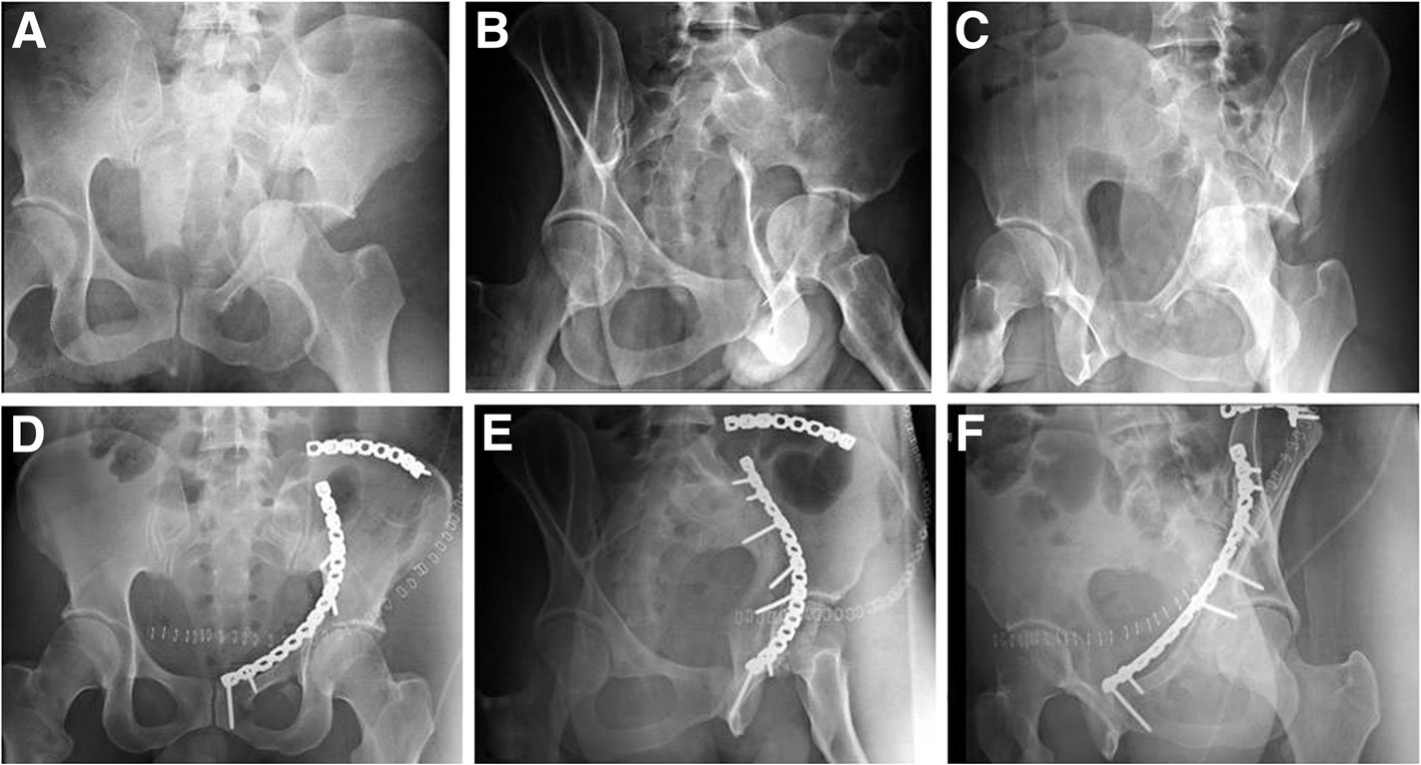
Example of an associated both column fracture treated through a modified ilioinguinal approach with two 3.5 mm reconstruction plates. (A-C) Preoperative X-rays (a.p., obturator oblique, iliac oblique). (D-F) Postoperative X-rays (a.p., obturator oblique, iliac oblique).
